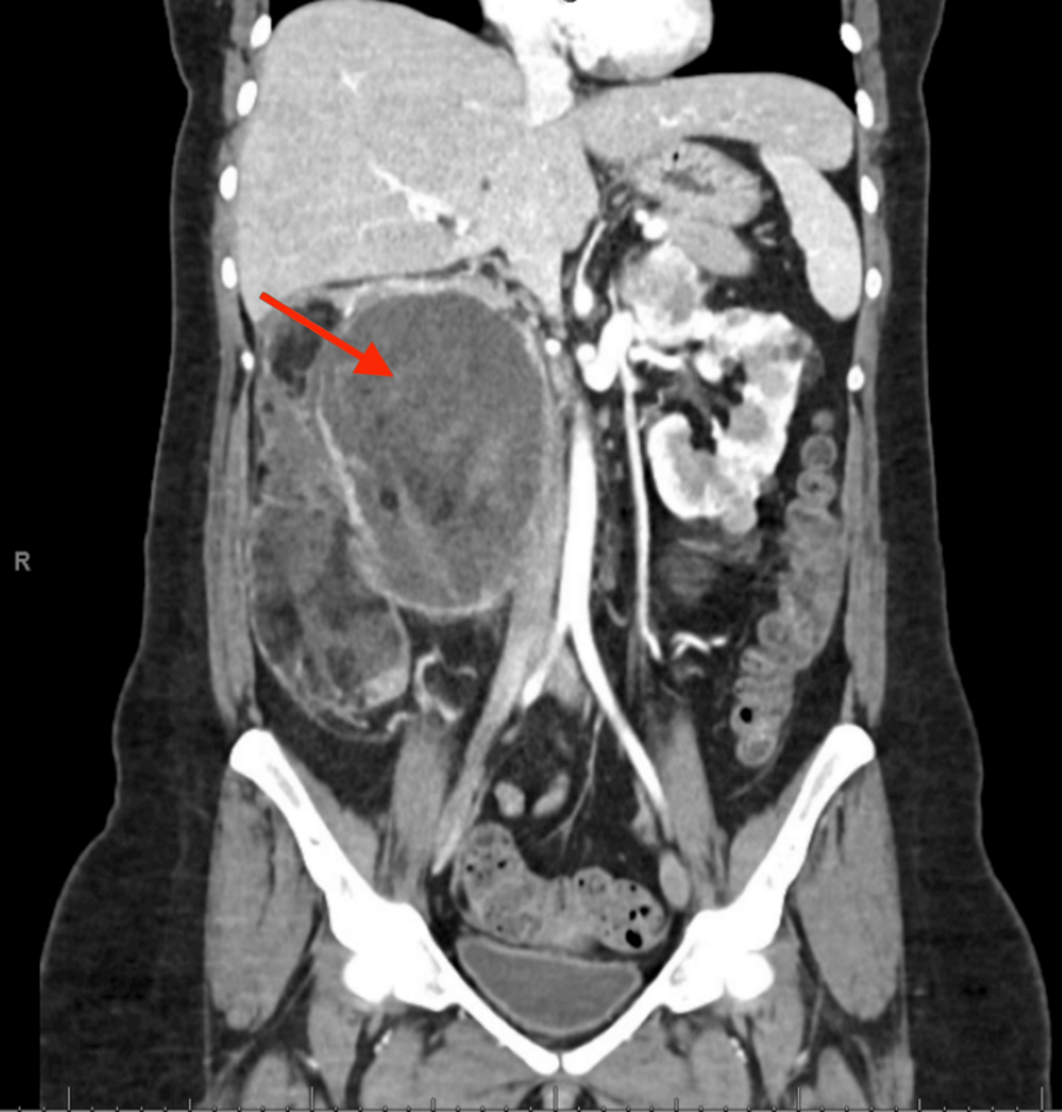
CT abdomen and pelvis: right renal angiomyolipoma measuring 16.1 x 12.0 x 19.6 cm in the coronal plane
